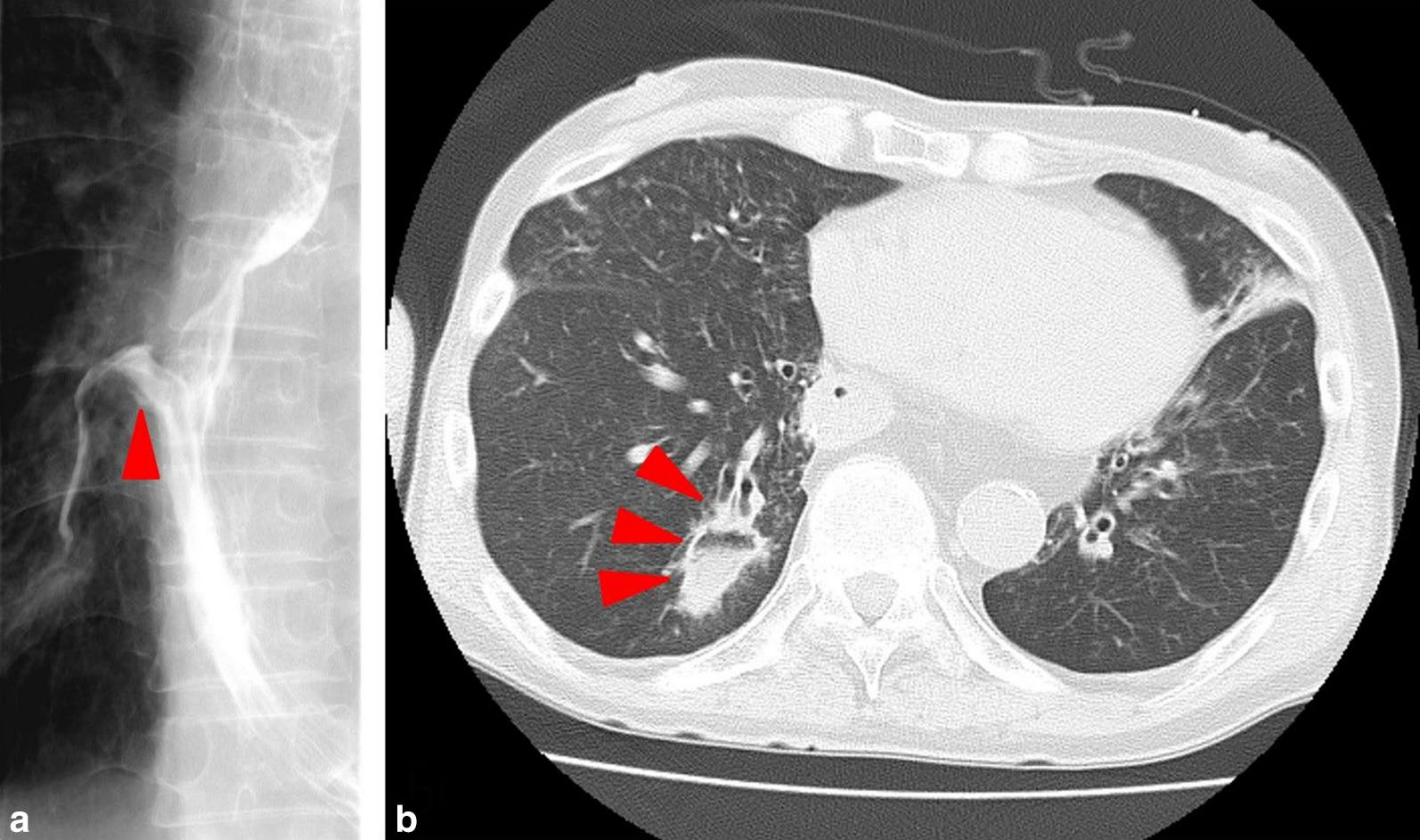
Imaging examinations performed after the conservative treatment. a A Gastrografin esophagram showed that the leakage was localized (red arrowhead). b CT showed an abscess as a reduction in permeability localized in the right lung (red arrowheads)
